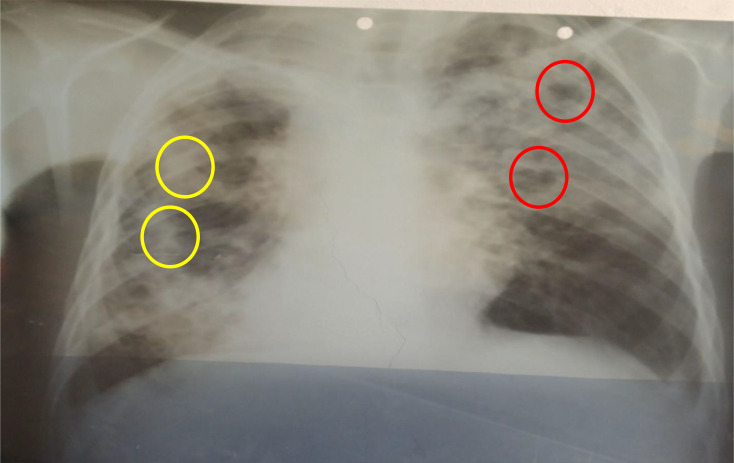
Chest X-ray of a patient diagnosed with advanced bilateral pulmonary tuberculosis Yellow indicates consolidation, and red indicates cavitation
